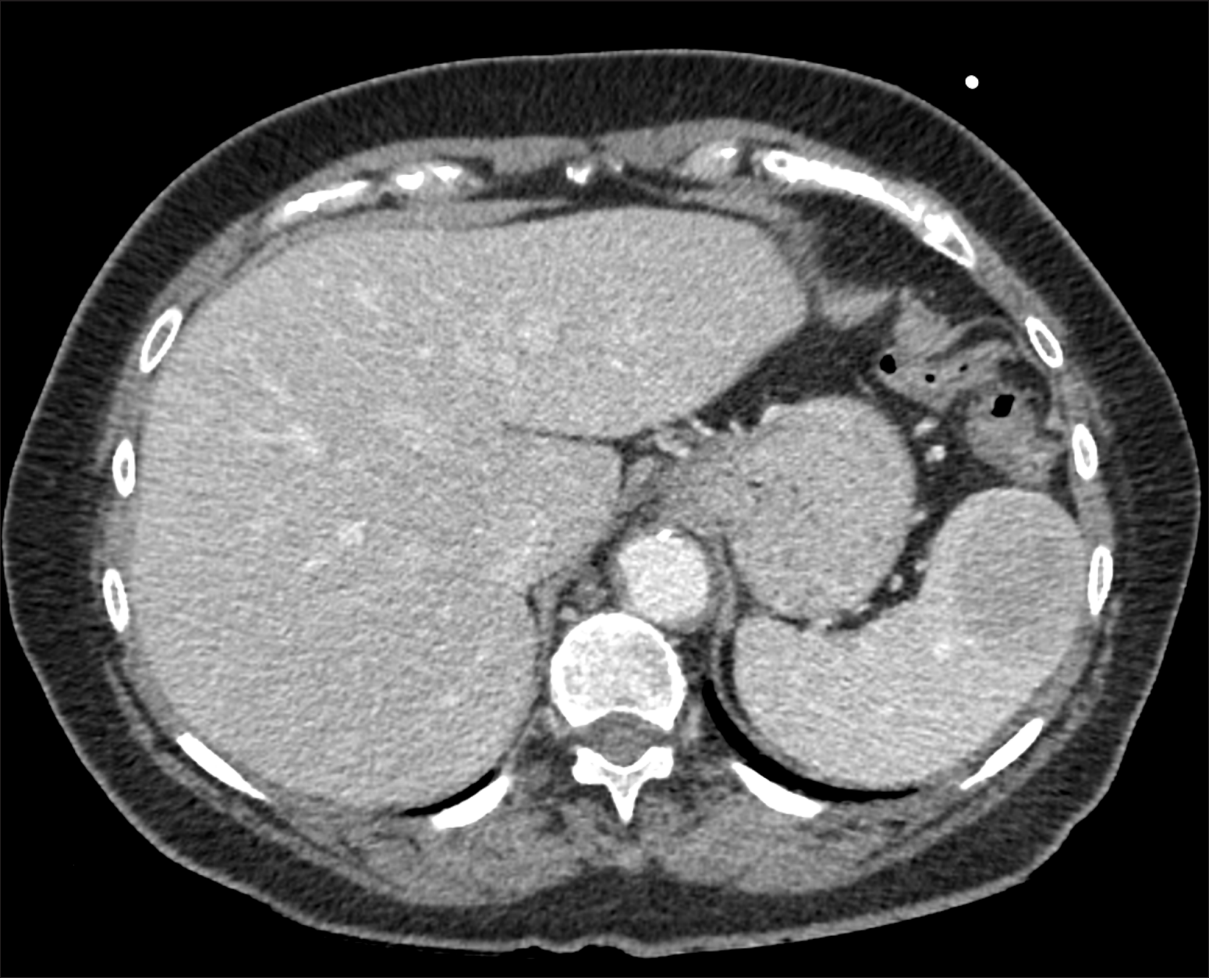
Axial Computed Tomography in the porto-venous phase depicts a sharply delineated lesion anteriorly in the spleen with relative hypo-enhancement compared to the surrounding splenic parenchyma.
